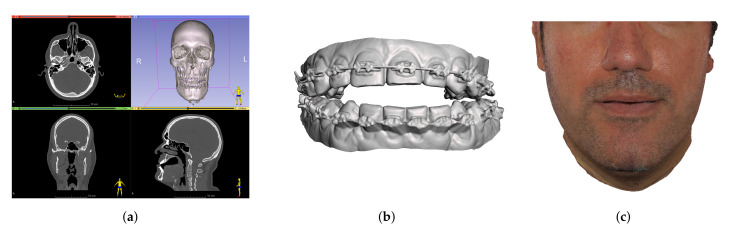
Summary of the patient imaging data collected for the planning process. CBCT-scan of the head (a), dental 3D scan (b), and textured face 3D scan (c) for patient M1.
